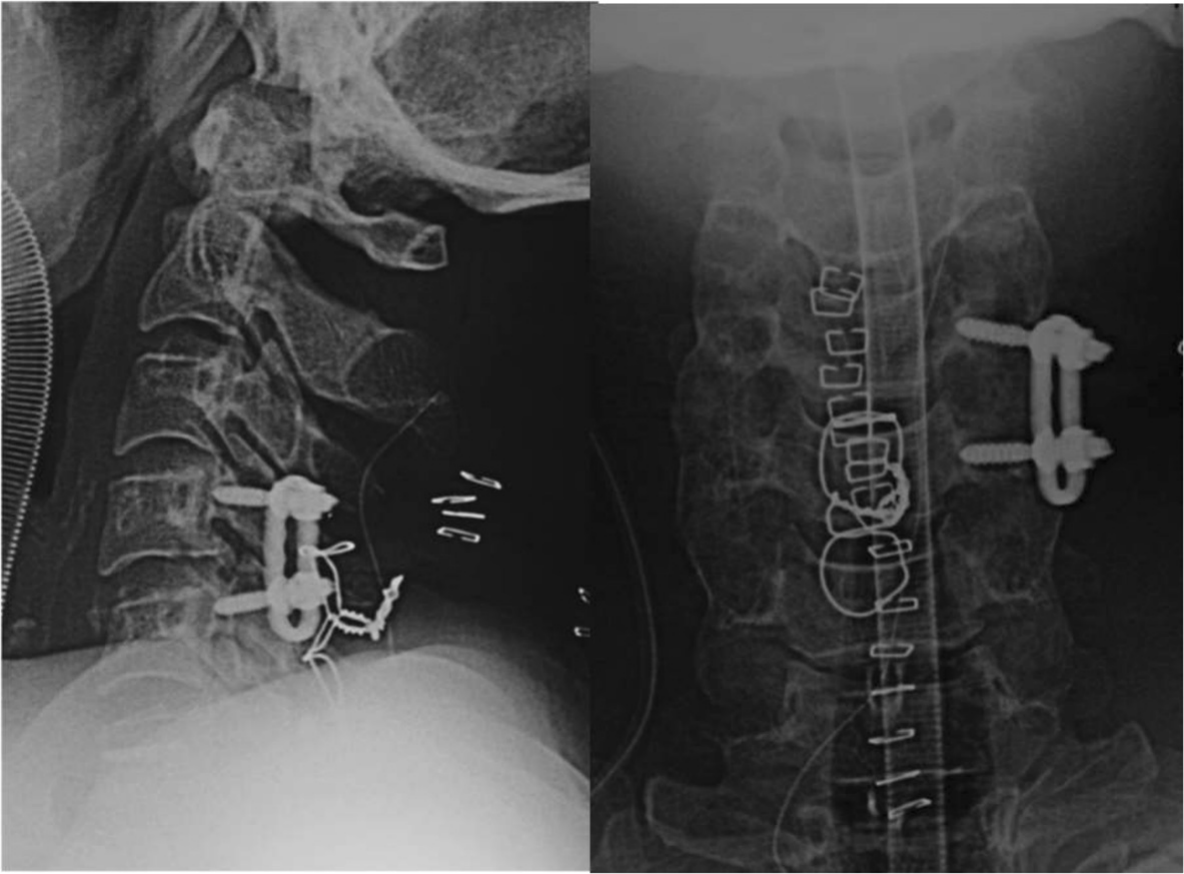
Postoperative radiography of the cervical spine.
